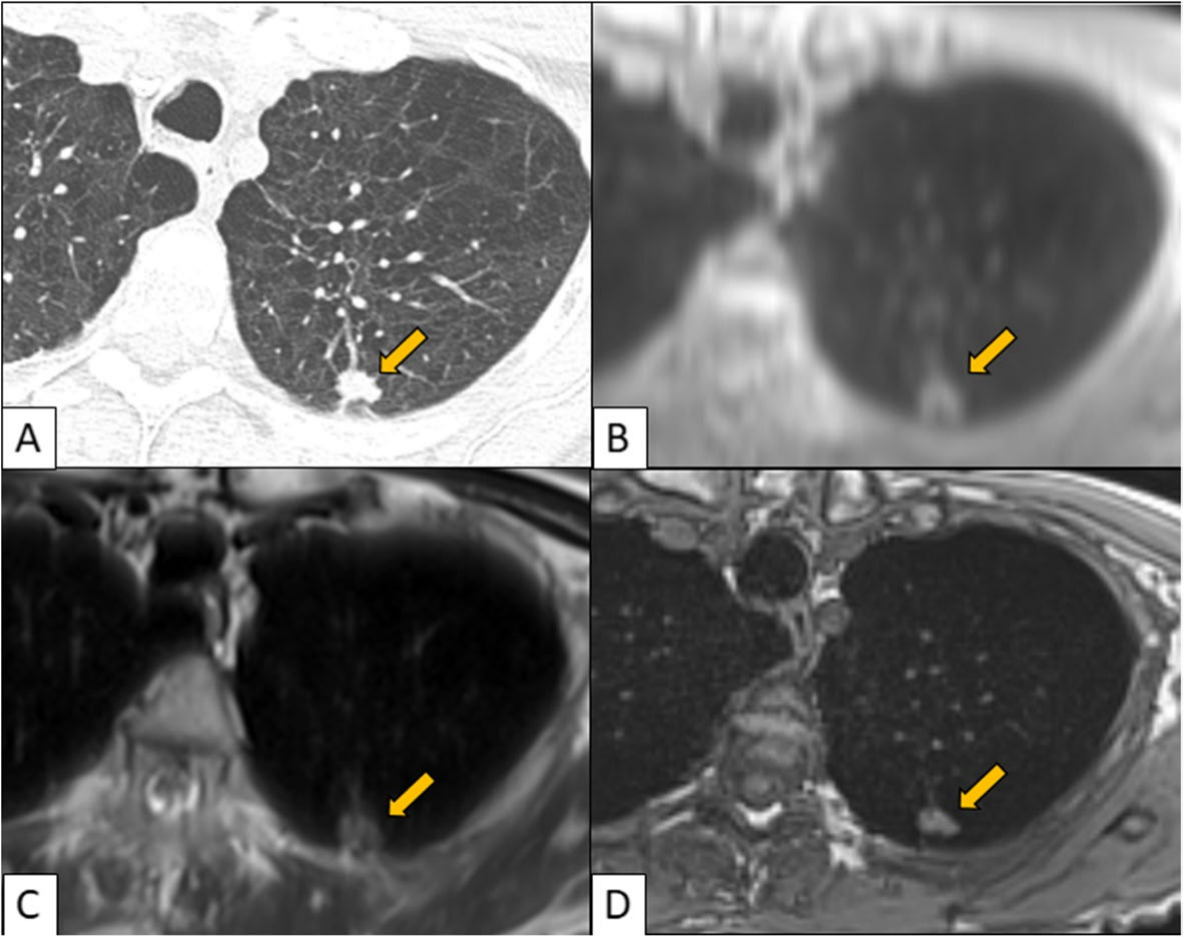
CT (A), UTE (B), HASTE (C), VIBE (D). Left upper lobe spiculated solid nodule is detected in a similar way in the 3 MR sequences: UTE, HASTE and VIBE
